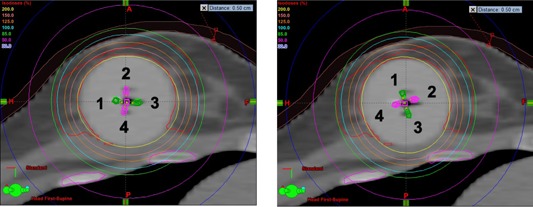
Comparison of isodose distribution between the original MDML plan (a) and a rotated plan (b) with 70° clockwise rotation for Case A. The isodose lines in (a) demonstrate an asymmetric dose distribution conformal to PTV_EVAL (red contour), while those in (b) show the unintended dose increase to skin and rib with less conformal dose to PTV_EVAL. The five multilumen catheters and their rotation are easily visualized in these two‐dimensional (2D) images at the central section of the balloon. In these 2D images, balloon‐to‐skin distance is larger than 0.5 cm. Note that the minimum balloon‐to‐skin distance can be accurately measured by a comprehensive review of 3D balloon relative to the skin in 3D planning CT images. For Case A, it was 0.5 cm and located in the distal (not central) section of balloon.
